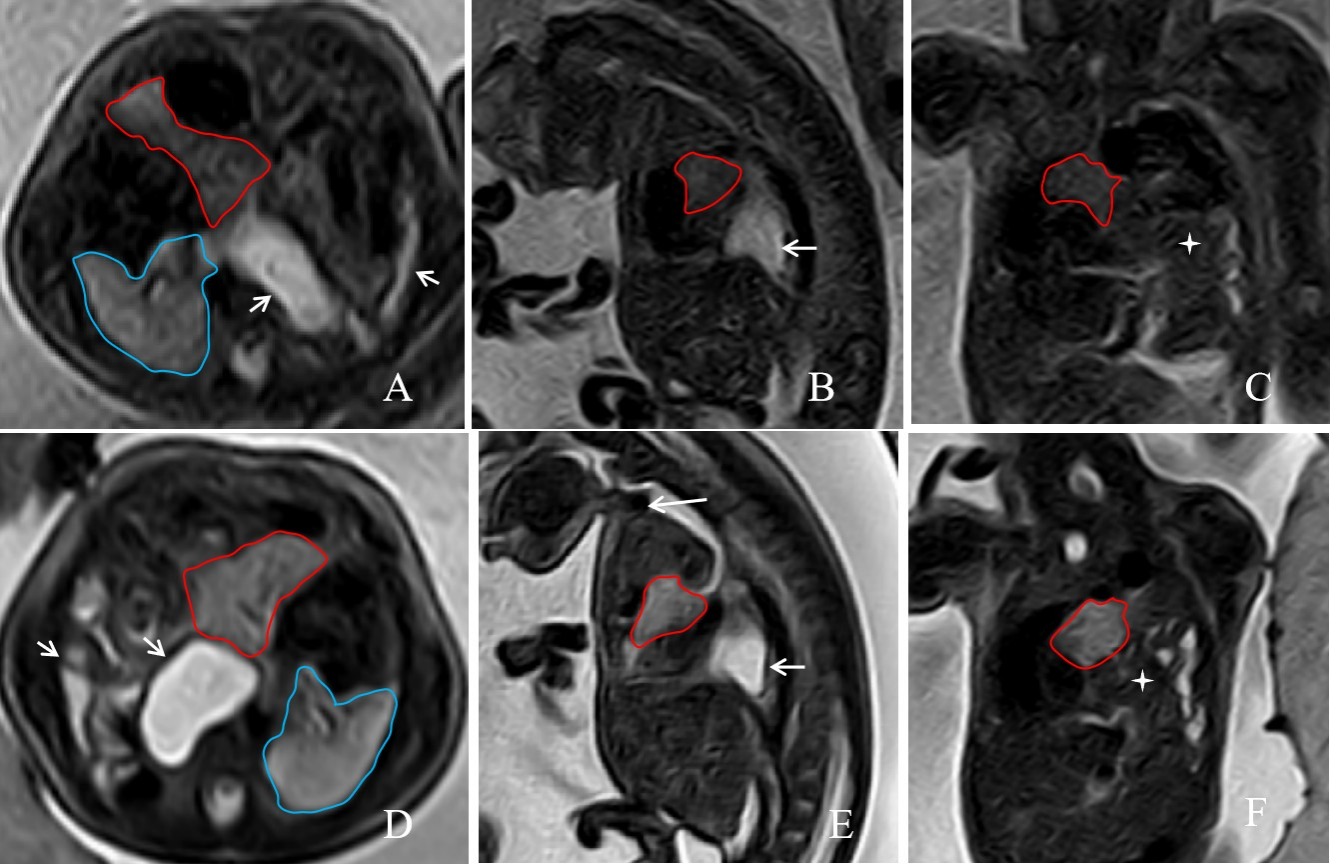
A 32-year-old pregnant woman, the fetus with left CHD on prenatal MRI. The axial, sagittal, and coronal T2-weighted images at 29w5d of gestation(A, B and C) before FETO treatment, and at 32w of gestation after FETO treatment(D, E and F) showed abdominal organs, such as the stomach, intestines, and partial left lobe of liver, herniated into the chest cavity(short arrow and cross Star). A sagittal T2-weighted image showed that a low-signal balloon was inserted into the trachea above the carina during FETO surgery(E, long arrow). The area delineated by the red line represents the left lung that has herniated into the chest cavity, while the area outlined by the blue line is the normal right lung.
